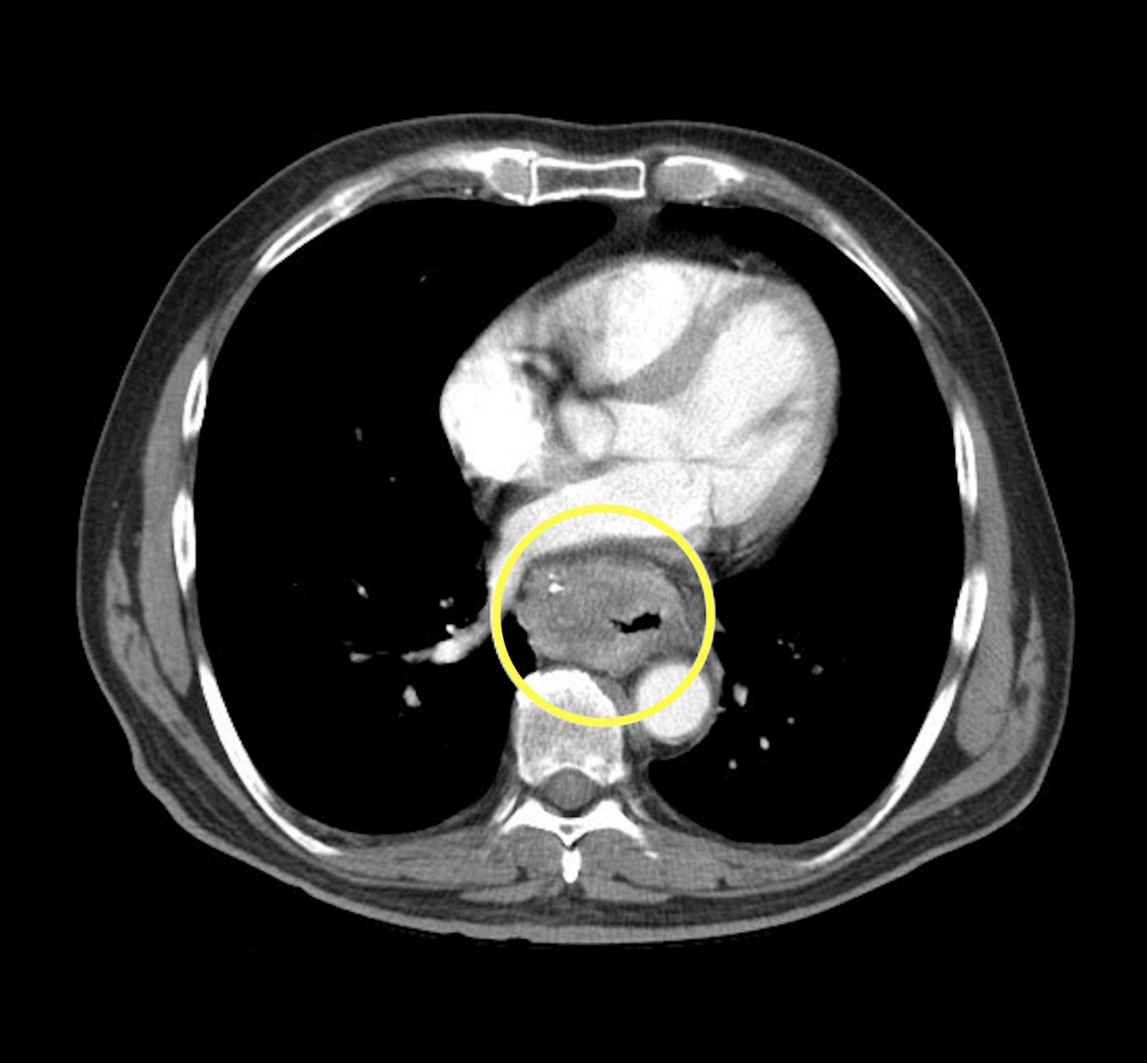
Axial contrast enhanced computed tomography (CT) chest (soft tissue window) demonstrating a solid heterogeneously enhancing esophageal mass (yellow circle).
